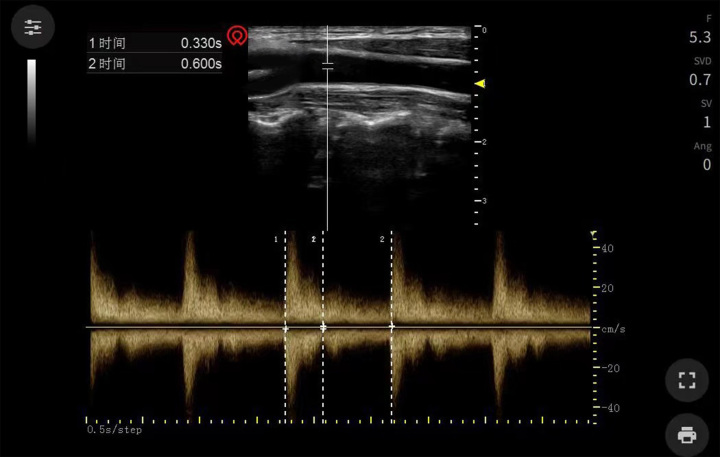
Carotid artery pulsed Doppler flow spectrograms.
